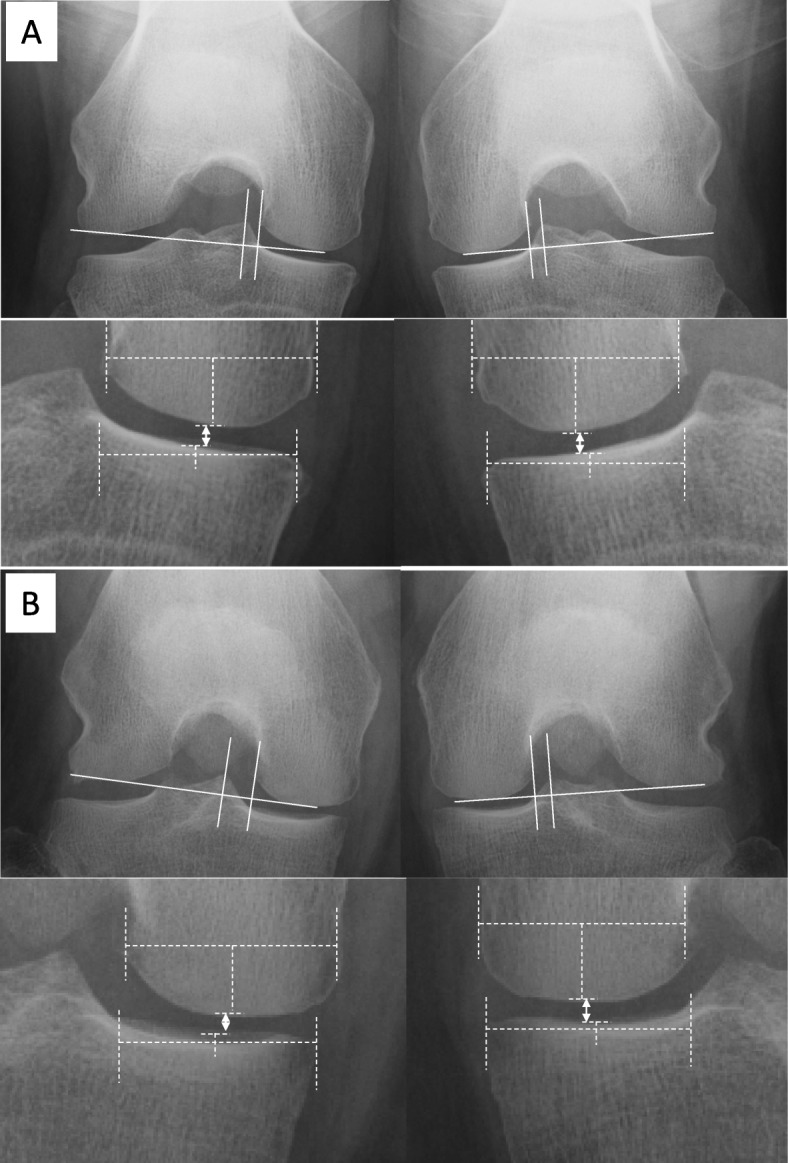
Representative posterior-anterior weight-bearing radiographs (Rosenberg view). a A 62-year-old woman was diagnosed with degenerative changes in the medial meniscus. The distance between medial tibial eminence to the medial femoral condyle (MTE–MFC distance): *right*, 3.3 mm; *left*, 3.1 mm. Medial joint space (MJS) width: *right*, 4.3 mm; *left*, 4.3 mm. b A 63-year-old woman was diagnosed with a right medial meniscus posterior root tear. MTE–MFC distance: *right*, 6.8 mm; *left*, 4.2 mm. MJS width: *right*, 2.8 mm; *left*, 4.3 mm. The *white double-headed arrows* indicate the MJS width
